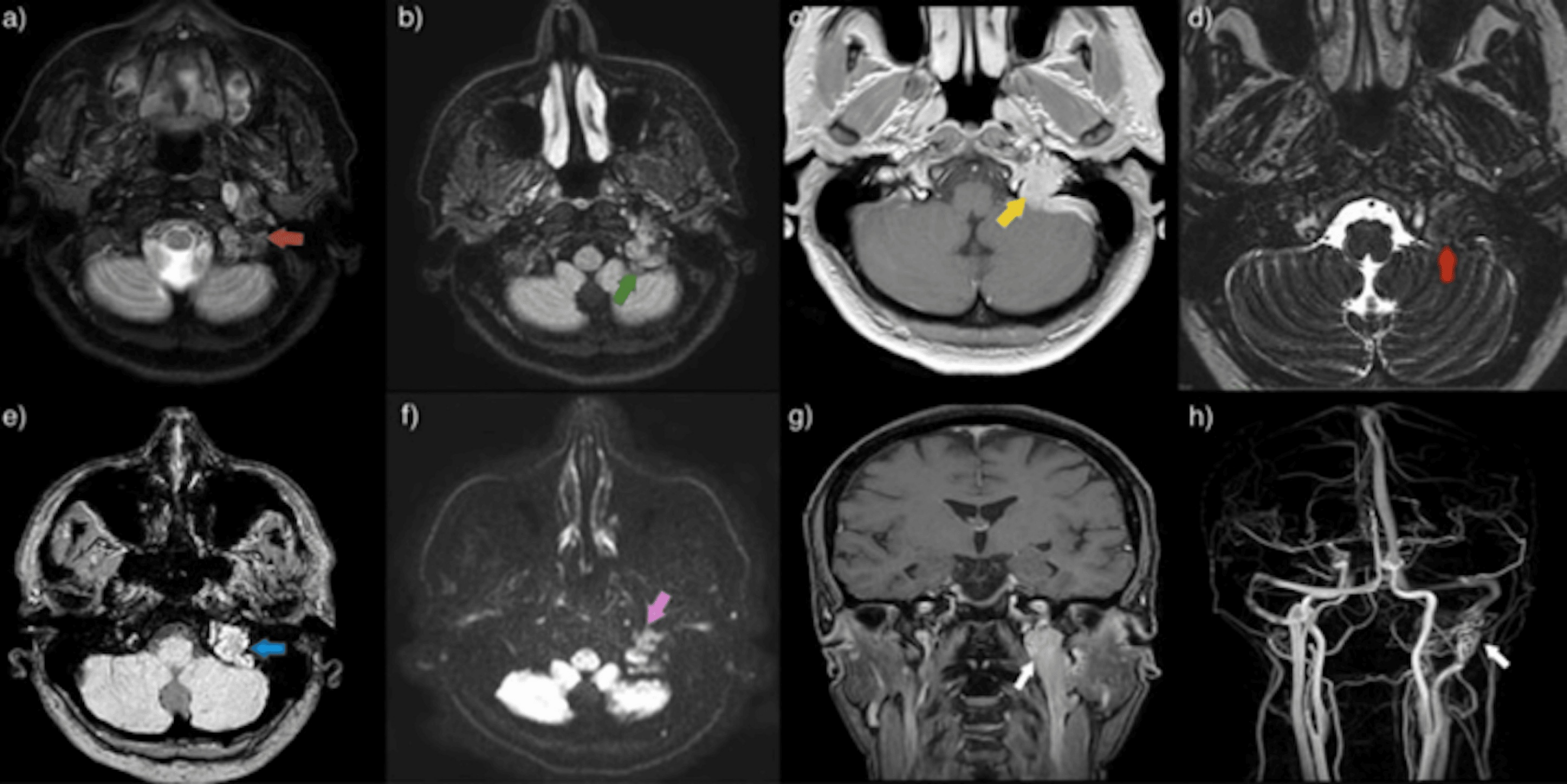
Mosaic of axial and angiographic magnetic resonance imaging sequences. Magnetic resonance imaging sequences, with and without gadolinium contrast, demonstrate a space-occupying lesion involving the left jugular foramen with bone erosion, prominent vascular structures arising from the external carotid artery, and venous drainage into an enlarged left internal jugular vein, consistent with a jugular paraganglioma (glomus jugulare). The lesion measures approximately 1.7 × 2.4 × 8.4 cm (anteroposterior × transverse × craniocaudal). (a) Axial T2-weighted fast spin-echo image showing an isointense lesion relative to cerebellar tissue at the level of the jugular bulb (orange arrow). (b) Axial T2-weighted FLAIR image showing the lesion at the left jugular bulb (green arrow). (c) Post-contrast T1-weighted gradient-echo image demonstrating avid enhancement of the lesion (yellow arrow). (d) Heavily T2-weighted axial image highlighting the lesion at the jugular bulb (red arrow) with a characteristic “salt-and-pepper” appearance due to flow voids from tumor hypervascularity. (e) Axial susceptibility-weighted (VenBOLD) image confirming the hypervascular lesion (light blue arrow). (f) Axial diffusion-weighted image revealing signal isointensity of the left jugular glomus (pink arrow). (g) Coronal post-contrast T1-weighted spin-echo image showing the lesion occupying the left jugular bulb (white arrow). (h) Three-dimensional phase-contrast angiographic reconstruction demonstrating hypervascularity of the lesion with arterial supply from branches of the external carotid artery (white arrow). FLAIR: fluid-attenuated inversion recovery
